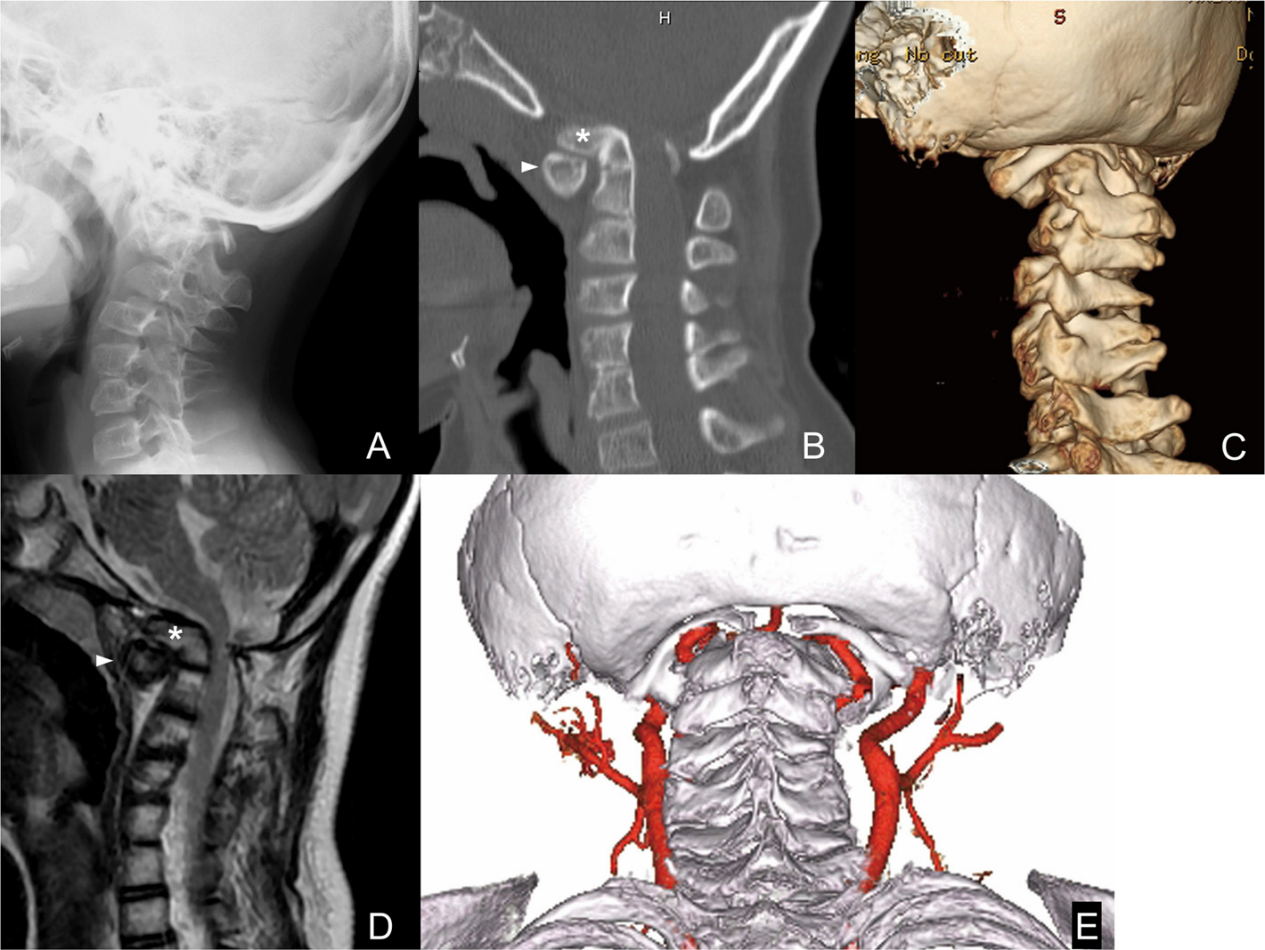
Preoperative imaging of the craniovertebral junction. (A) Lateral radiography shows malalignment of the craniovertebral junction. (B) Midsagittal reconstruction from computed tomography shows an anteriorly tilted odontoid process of C2 and migration of a hypoplastic C1 posterior arch into the foramen magnum. (C) Posterolateral view of three-dimensional computed tomography shows migration of the C1 posterior arch into the foramen magnum and rachischisis (spina bifida) of the C1 posterior arch. (D) Midsagittal T2-weighted magnetic resonance imaging shows compression of the spinal cord by the basilar invagination and an anteriorly shifted C1 posterior arch. (E) Posteroinferior view of three-dimensional computed tomography angiography shows anomalous courses of bilateral vertebral arteries (persistent first intersegmental artery), which were located between the C1 posterior arch and the pars interarticularis of C2. Asterisks, odontoid process; arrowheads, C1 anterior arch.
